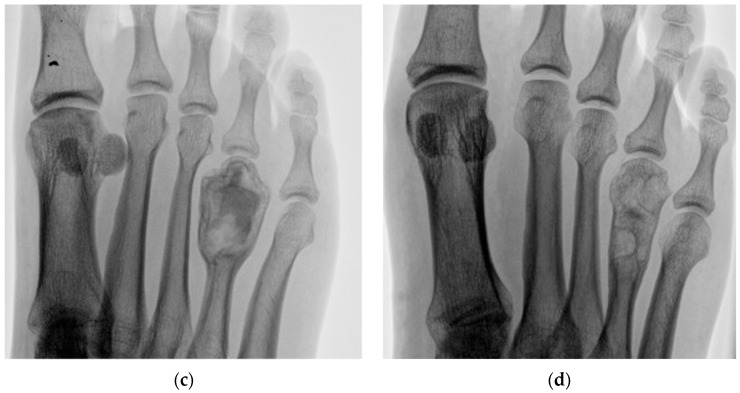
Dorsoplantar (DP) radiographs of right foot (*Case 1*): (a) preoperatively, showing an aneurysmal bone cyst in the distal fourth metatarsal; (b) immediately postoperatively, following excision and curettage, with the contained defect filled with GeneX; (c) at 6 months follow-up, the GeneX shows partial resorption and there is progression of bone healing with cortical definition maintained; and (d) at 12 months follow-up, the GeneX has been completely resorbed and there is evidence of significant bone remodelling.
